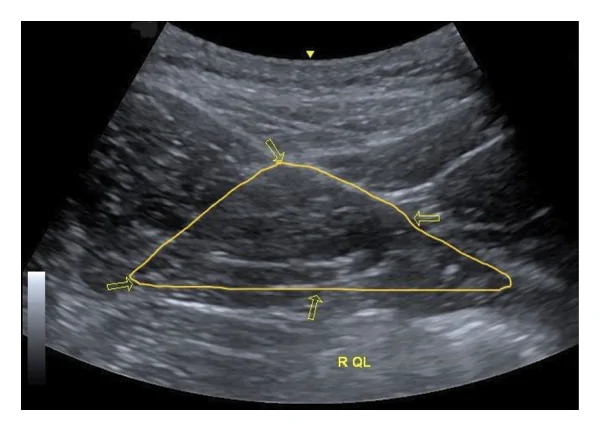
Ultrasound image of the right quadratus lumborum cross‐sectional area.
